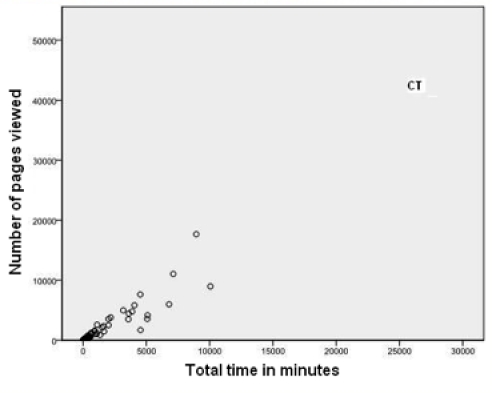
Number of pages viewed versus total time in minutes for all participants. This shows the Caretaker (CT) (with over 25,000 minutes logged on and over 40,000 pages viewed) as an outlier
